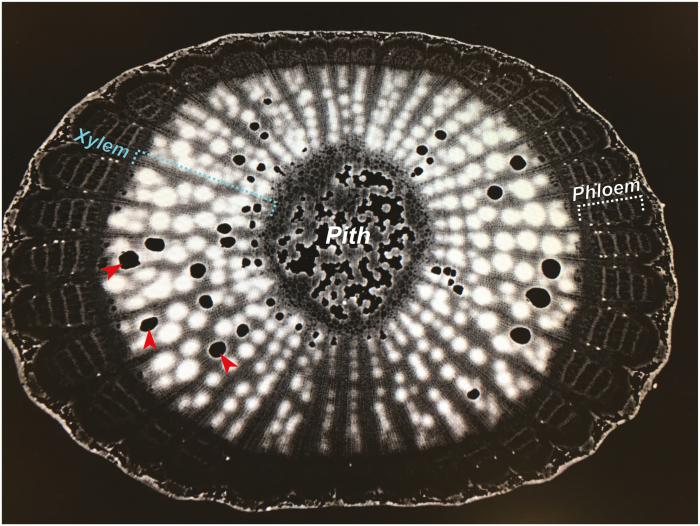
Non-invasive visualization of embolisms in a 1-year-old grapevine cane using high-resolution computed tomography. Functional xylem vessels appear bright white (visualized via the contrasting agent iohexol), but some vessels have become embolized (black xylem vessels, examples shown with red arrowheads) and do not conduct water.
